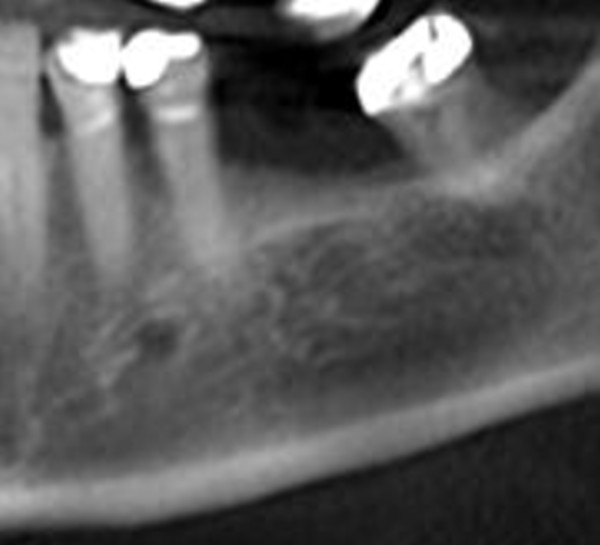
Orthopantomogram is showing no bony destruction
